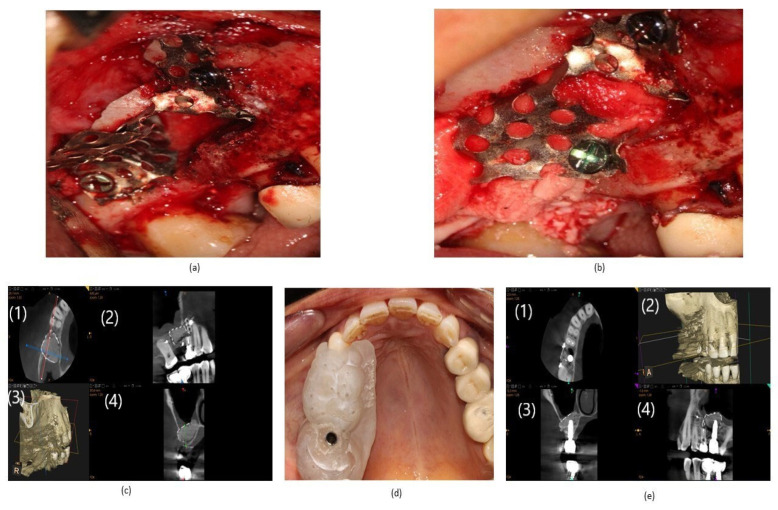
(a) Design of the customized titanium mesh formwork fixed inside the patient’s maxillary sinus. (b) Formwork with collagen membrane and biomaterial before closing the formwork window. (c) The 5 × 5 oblique CBCT slice prior to implant placement, showing the absence of sinus pathology and bone gain. (1) CBCT coronal slice; (2) CBCT frontal slice; (3) 3D reconstruction; (4) CBCT sagittal slice. (d) Prosthetically guided implant placement using a previously fabricated surgical guide. (e) Two-year follow-up CBCT showing implant continuity and the absence of sinus pathology. (1) CBCT coronal slice; (2) 3D reconstruction; (3) CBCT sagittal slice; (4) CBCT panoramic frontal slice.
